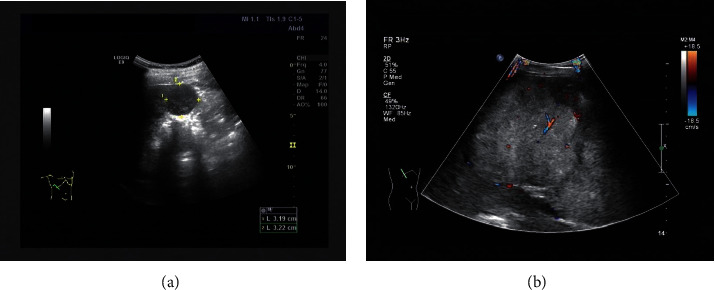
The color Doppler ultrasound manifestation of hepatocellular carcinoma. (a) The patient was a 58-year-old male with a history of hepatitis B. Two-dimensional ultrasound of the liver shows that 3.2*∗*3.2 cm hypoechoic nodules were visible in the liver, and the boundary was still clear. (b) Blood flow signals can be seen inside and around the mass. Pulse Doppler showed that the blood flow was high speed and high impedance, RI: 0.83.
